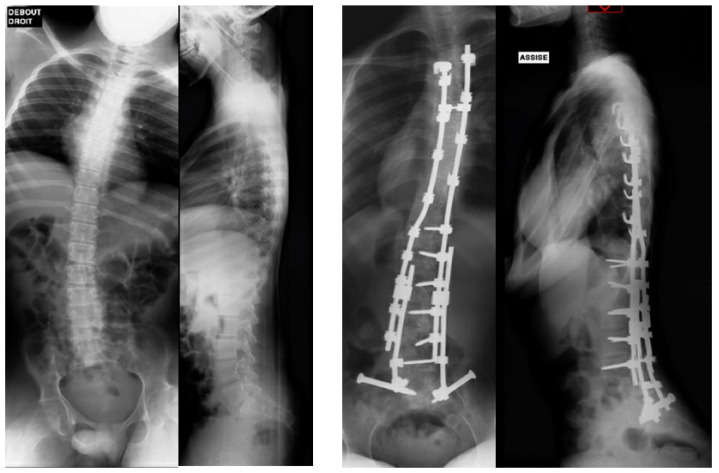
Pre- and postoperative X-rays after posterior spinal fusion.
